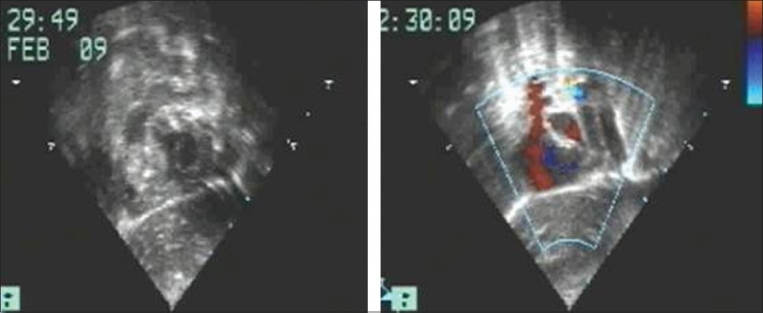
Echocardiogram in subcostal saggital view showing presence of the right ventricular mass
